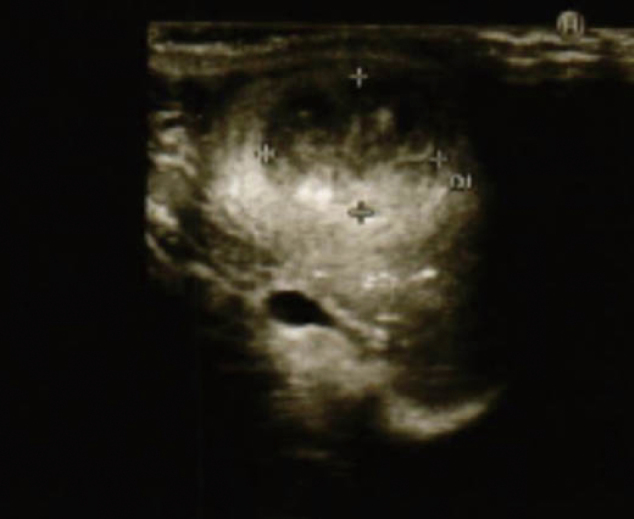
Ultrasound reveals heterogeneous neoplastic lesion in the right testis
